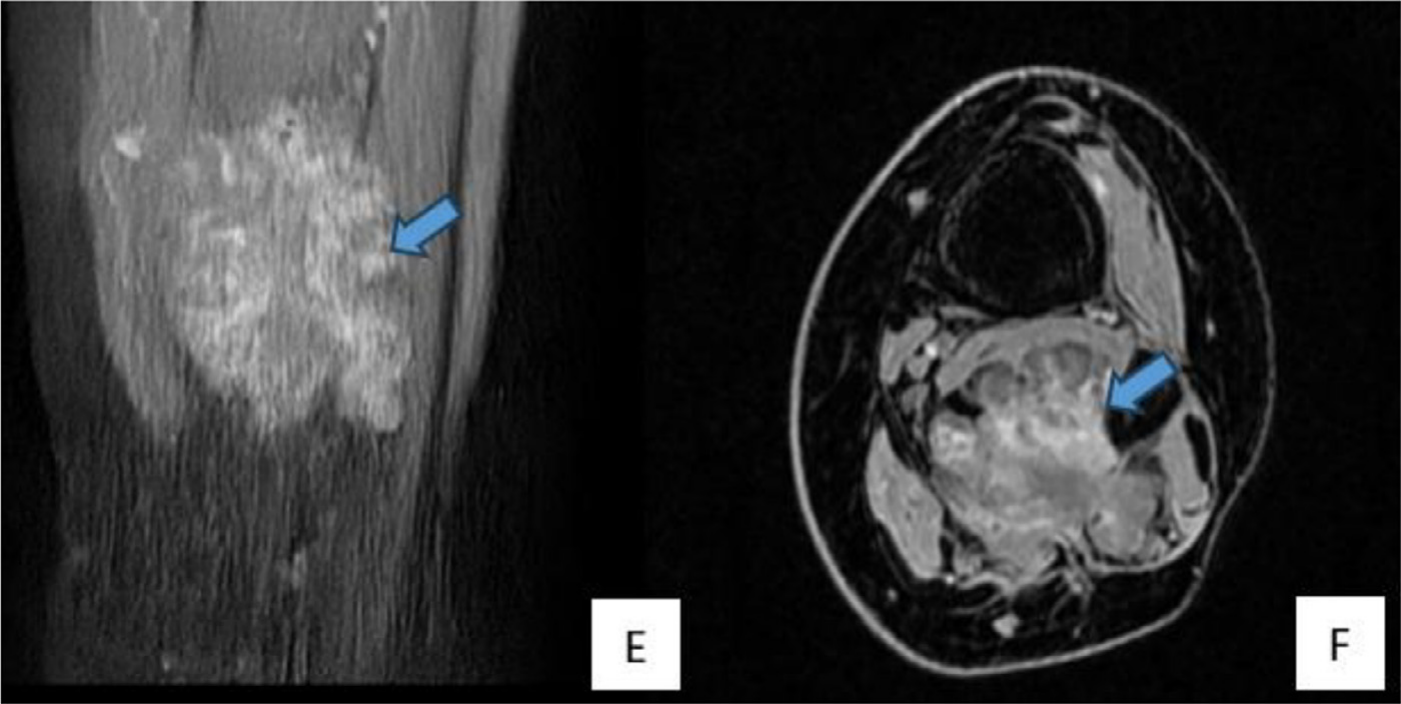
(E) Coronal and (F) axial postcontrast T1-weighted images demonstrating early and intense enhancement of the lesion following intravenous gadolinium administration, consistent with its vascular nature.
